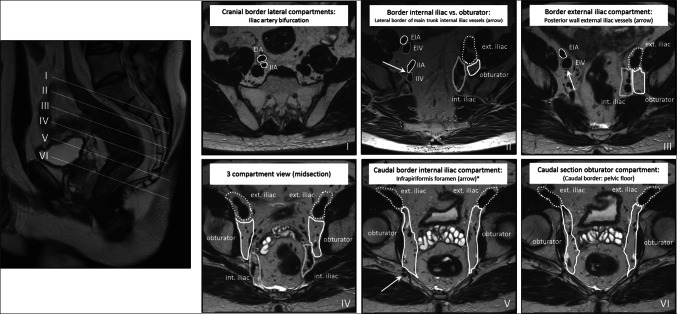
Anatomical boundaries of lateral lymph node stations (external iliac, internal iliac, obturator) on MRI. EIA = external iliac artery, EIV = external iliac vein, IIA = internal iliac artery, IIV = internal iliac vein. The border between the internal iliac and obturator compartments is defined by the lateral border of the main trunk of the internal iliac vessels (II–IV). The posterior wall of the EIV defines the border between the external iliac and obturator plus internal iliac compartments (II–VI). *The infrapiriformis foramen represents the transit point of the internal iliac vessels from the internal iliac compartment into the pudendal canal (V). This figure is largely based on a map previously published by Ogura et al JAMA Surg 2019;254: e192172 (supplement) [26]
